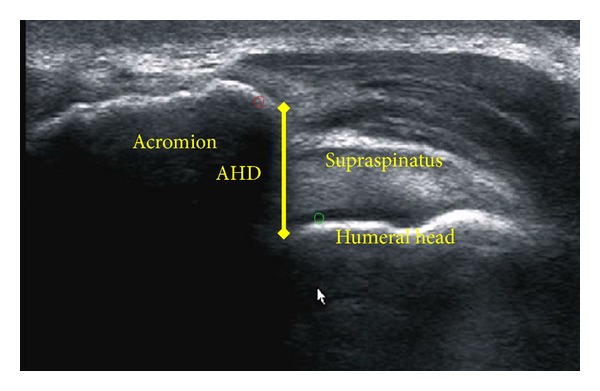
Ultrasonographic image of the acromiohumeral distance (AHD).
